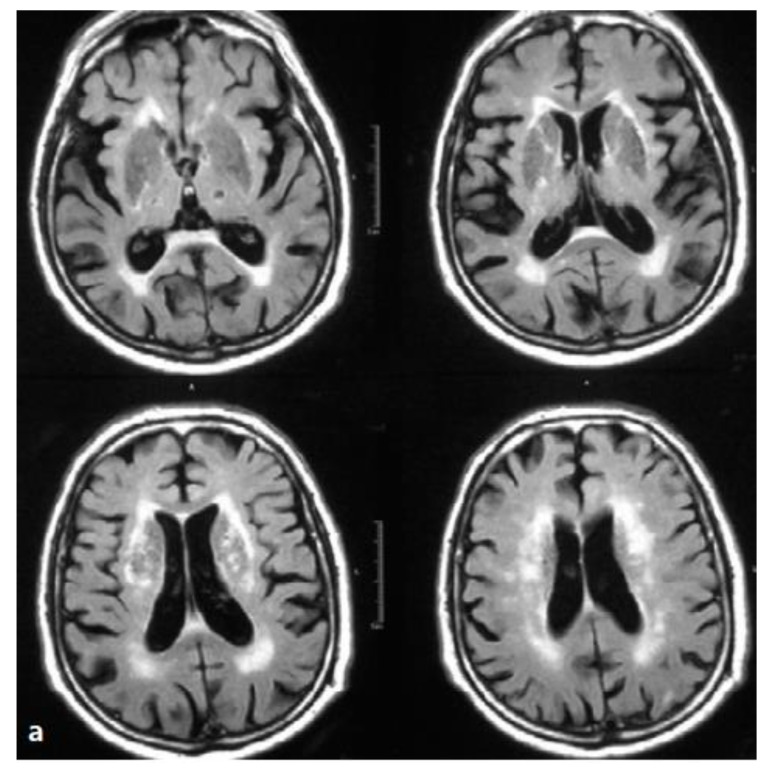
Brain MRI. Axial T2-weighted scans and fluid attenuation inversion recovery (FLAIR). Hypertension and recurrent *strokes* with subcortical lesions. Wide alteration of periventricular and lobar white matter signal. The image shows lacunar lesions involving thalami and internal capsules (lacunar state), together with the so-called “cribrose state”, with marked dilatation of base nuclei perivascular spaces.
